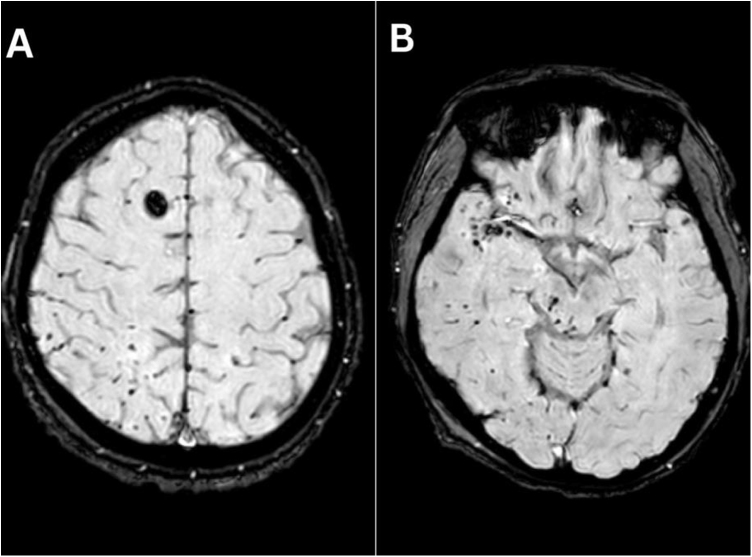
MRI brain, SWI, (A) showing multiple hypointense lesions throughout the cerebral cortex, (B) showing multiple hypointense lesions in the brainstem and cerebral cortex.
